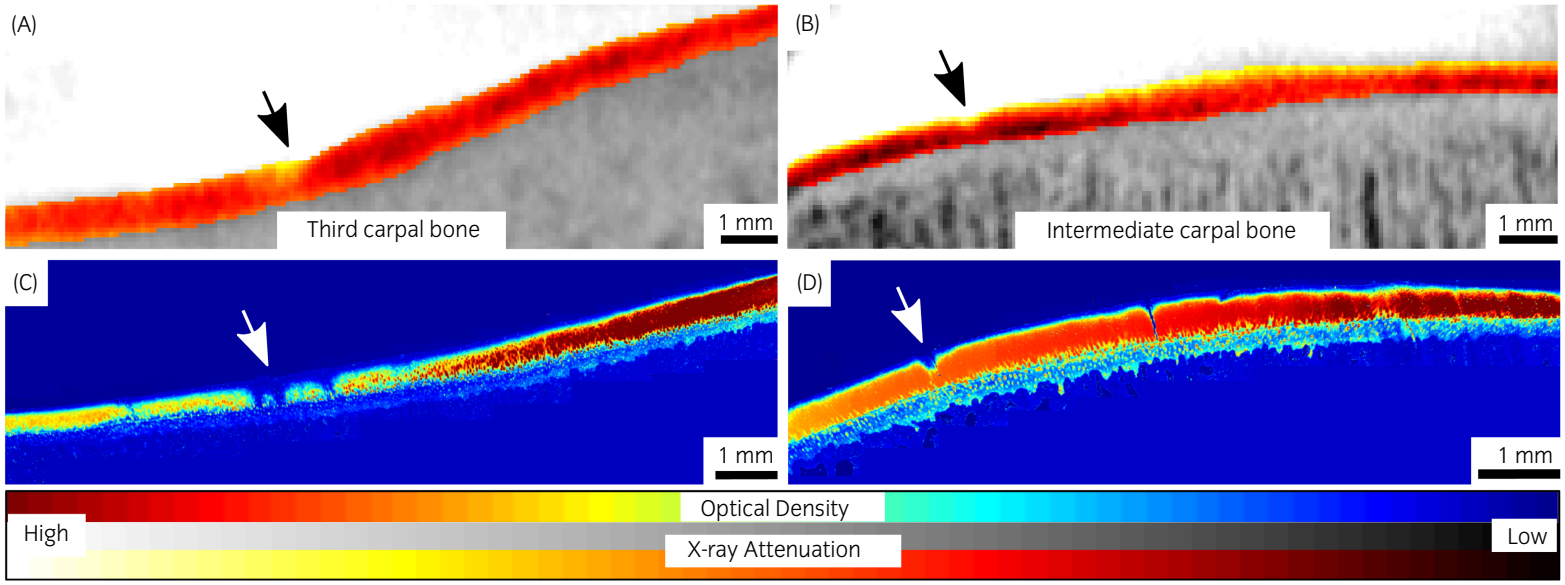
Computed tomography (A and B) and optical density maps (C and D) of two ponies having sharp grooves (black and white arrows) on the articular surfaces of the third carpal bone and the intermediate carpal bone. Computed tomography maps, acquired at the 60 minutes time point, show a degeneration‐related increase in ioxaglate diffusion into the cartilage at the grooved region and immediately adjacent to this. Similarly, proteoglycan loss is visible in the optical density maps
